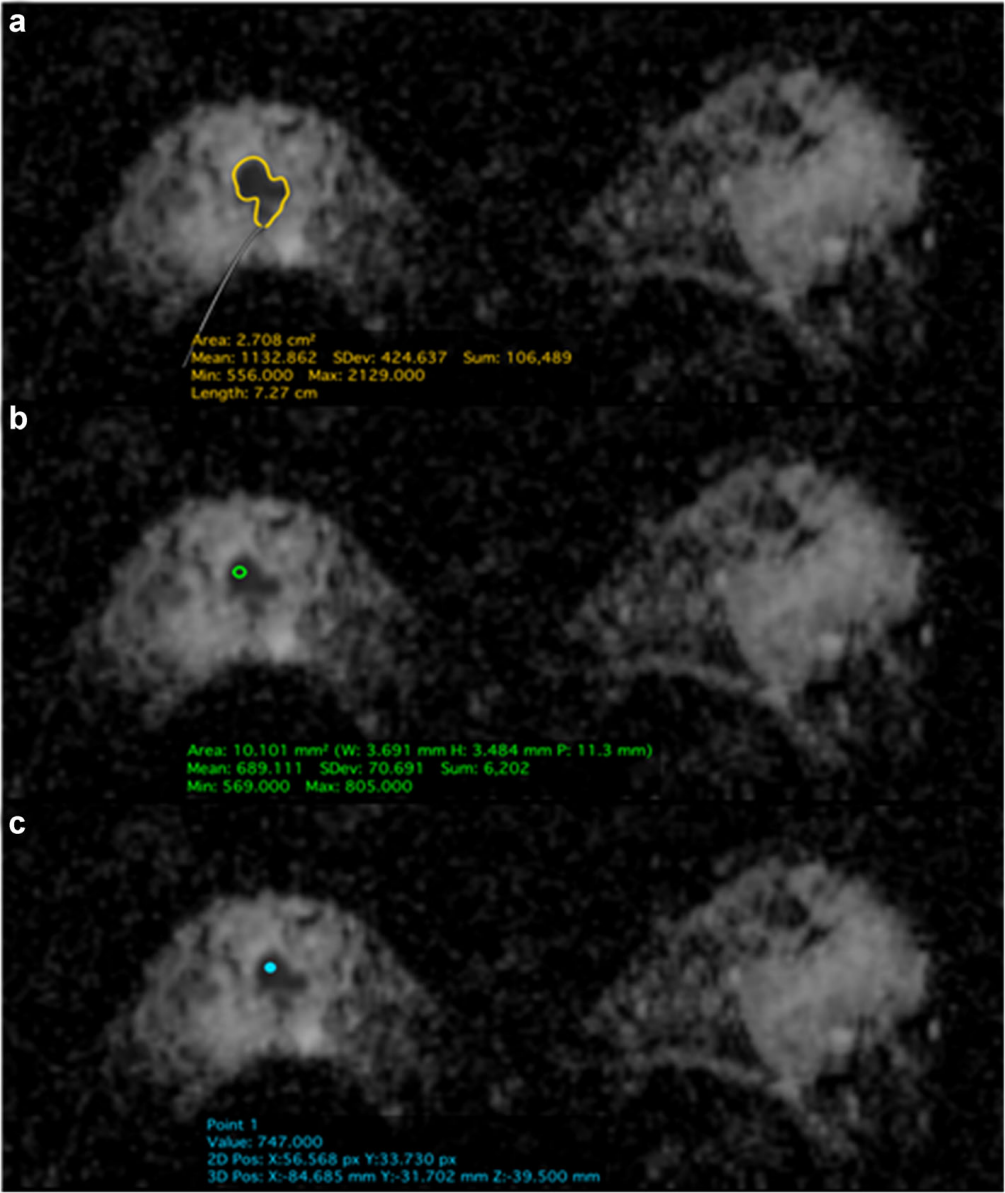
Invasive ductal carcinoma, grade 2, in a 47-year-old patient undergoing preoperative MRI. Apparent diffusion coefficient (ADC), axial views (a–c). The images show a hypointense area of restricted diffusion in the central part of the right breast. Examples of the three methods used to measure the ADC values: a whole tumor delineation, b darkest part of the tumor delineation, and c point tumor delineation. The three regions of interest show low ADC values < 1.3 × 10^−3^ mm^2^/s indicating that the enhancement is highly suspicious of malignancy (Breast Imaging Reporting and Data System 5)
